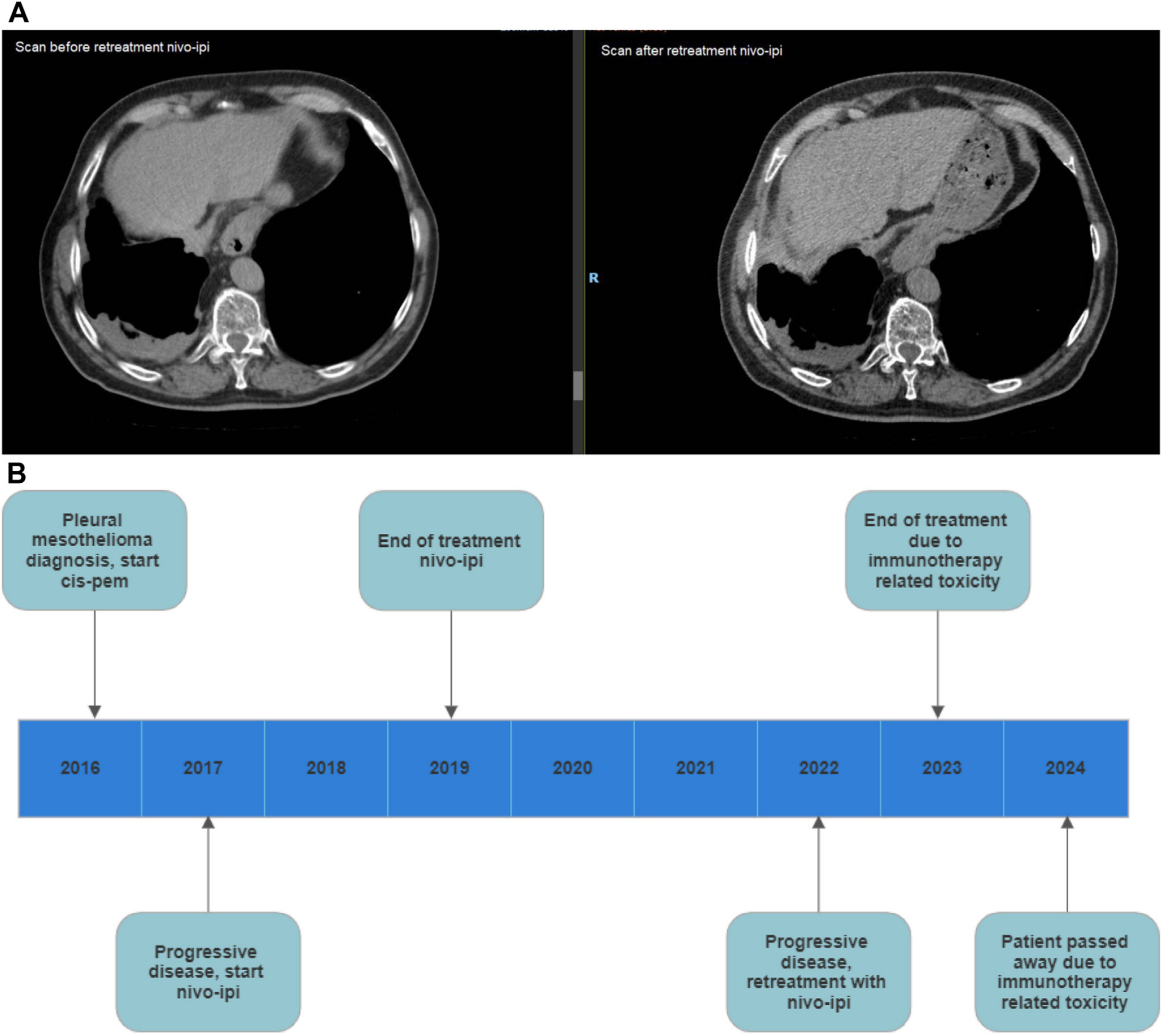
(*A*) CT scan (without contrast) 1 week before start of retreatment with nivo-ipi (left) and CT scan (without contrast) 6 weeks after start of retreatment with nivo-ipi (right). (*B*) Timeline of events. Cis-pem, cisplatin-pemetrexed; CT, computed tomography; nivo-ipi, nivolumab-ipilimumab.
